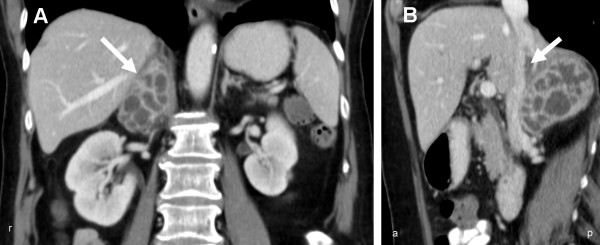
(A) Contrast-enhanced Coronal CT image of the abdomen shows a 6 cm large suprarenal, contrast enhancing tumour with central septet necrosis (→) (B) Coronal image shows infiltration of the Vena cava inferior and inside a 3-4 cm long thrombus (→). In addition an infiltration of the hepatic and renal capsule as well as the diaphragm is shown.
